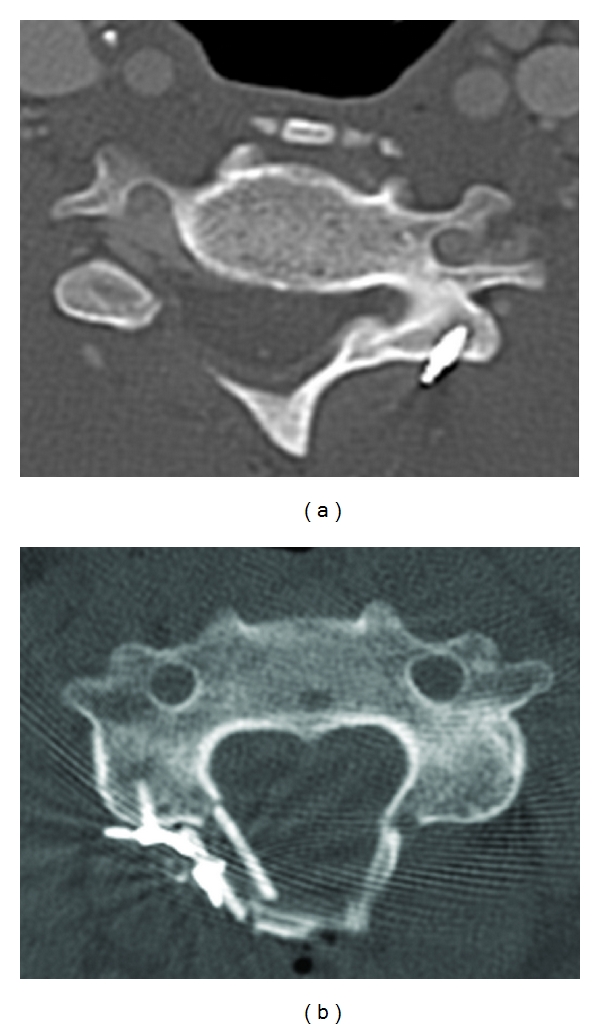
Axial CT scans of patients following expansive open-door laminoplasty. The laminar opening has been maintained with suture anchors (a) and miniplate fixation (b).
